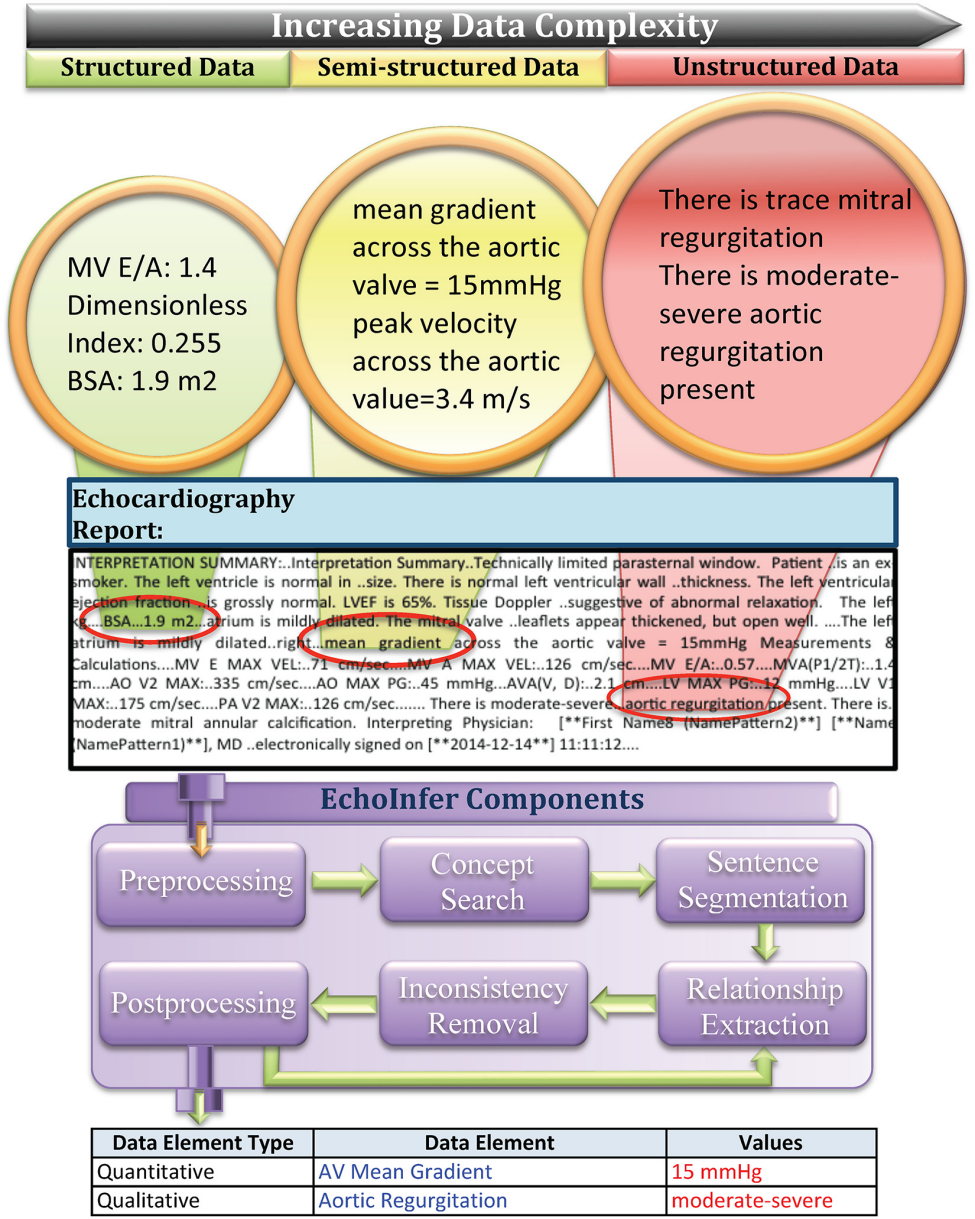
Extraction of data elements and values into structured format from structured, semi-structured, and unstructured data from echocardiography reports.
